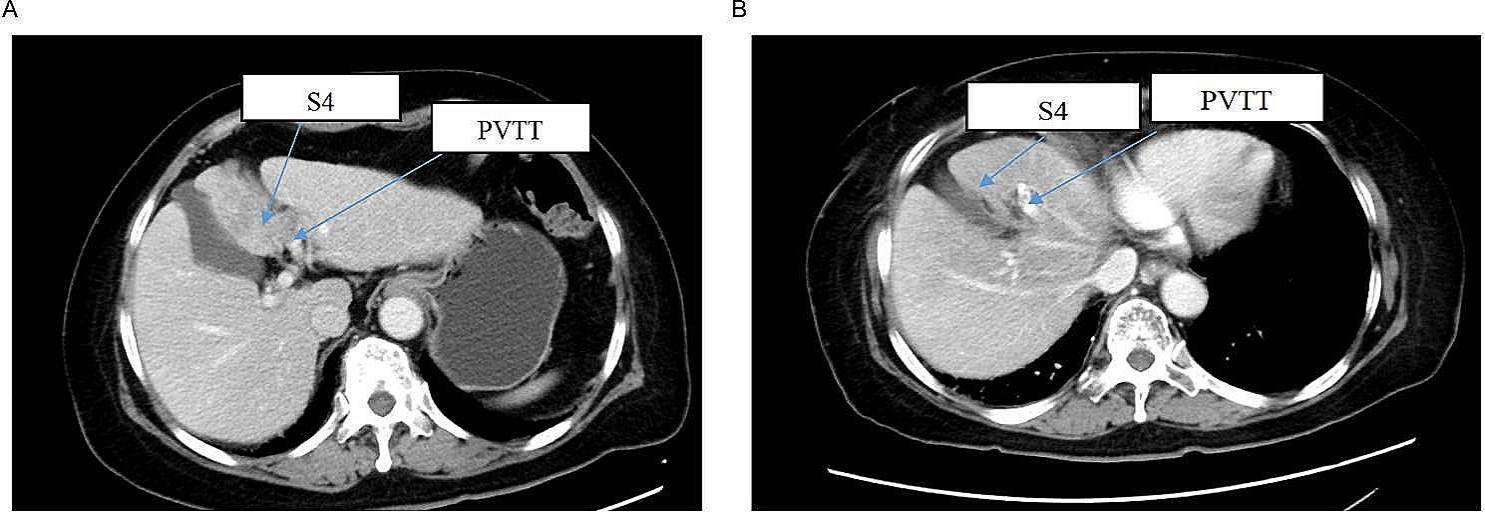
Representative sequential MRI images for one patient. (A) The images show the primary tumor and PVTT before RT treatment. (B) The images show the primary tumor and PVTT after RT treatment MRI: magnetic resonance imaging; PT: primary tumor; PVTT: portal vein tumor
